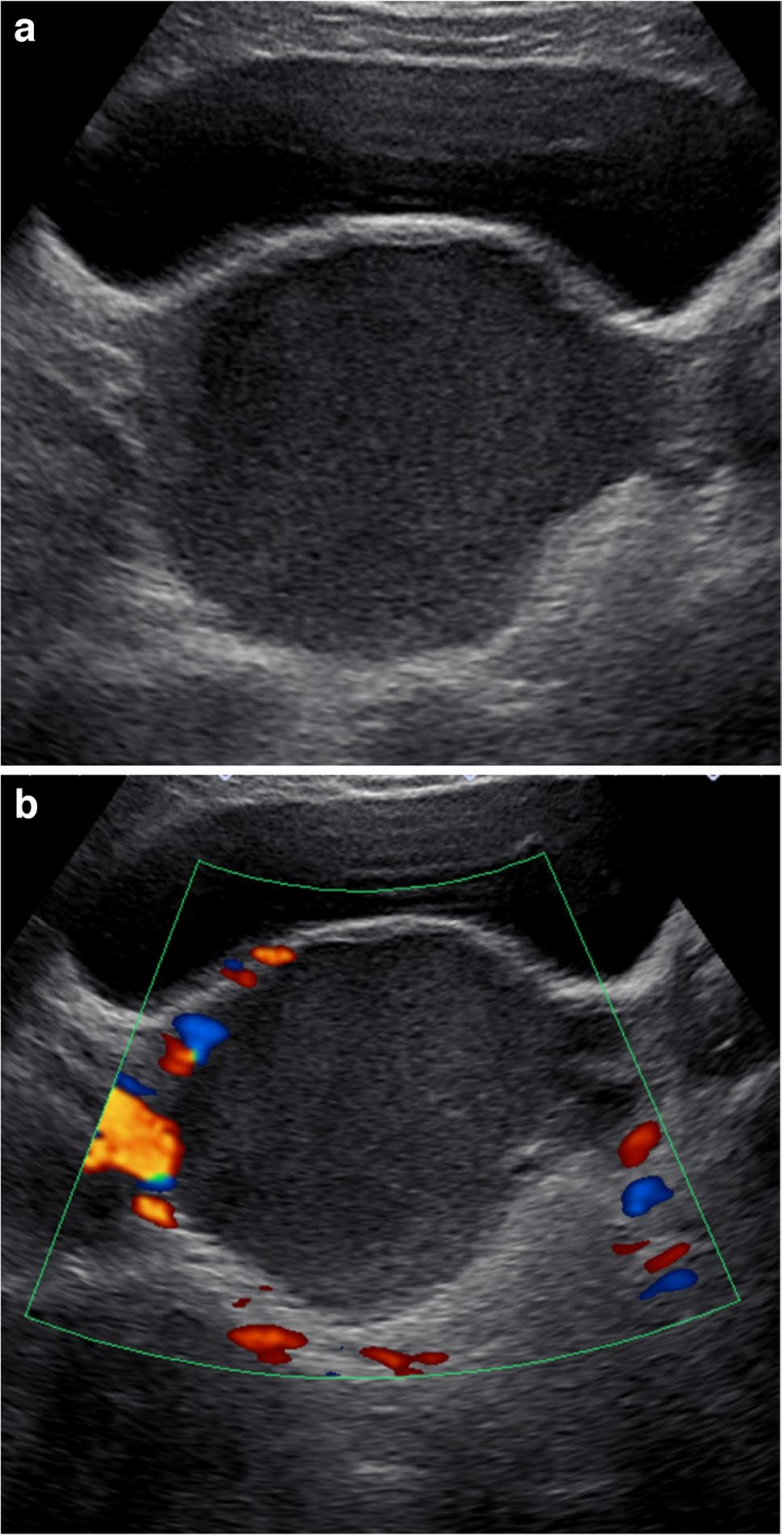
Ultrasound image example of a classic benign lesion, O-RADS 2, which all 3 readers labeled correctly in a 17-year-old female: Transverse static grayscale (a) and color Doppler (b) ultrasound images demonstrates a 8.9-cm right ovarian cystic lesion with homogenous low-level echoes without internal vascularity. Ultrasound findings are typical for an endometrioma, which was proven pathologically
